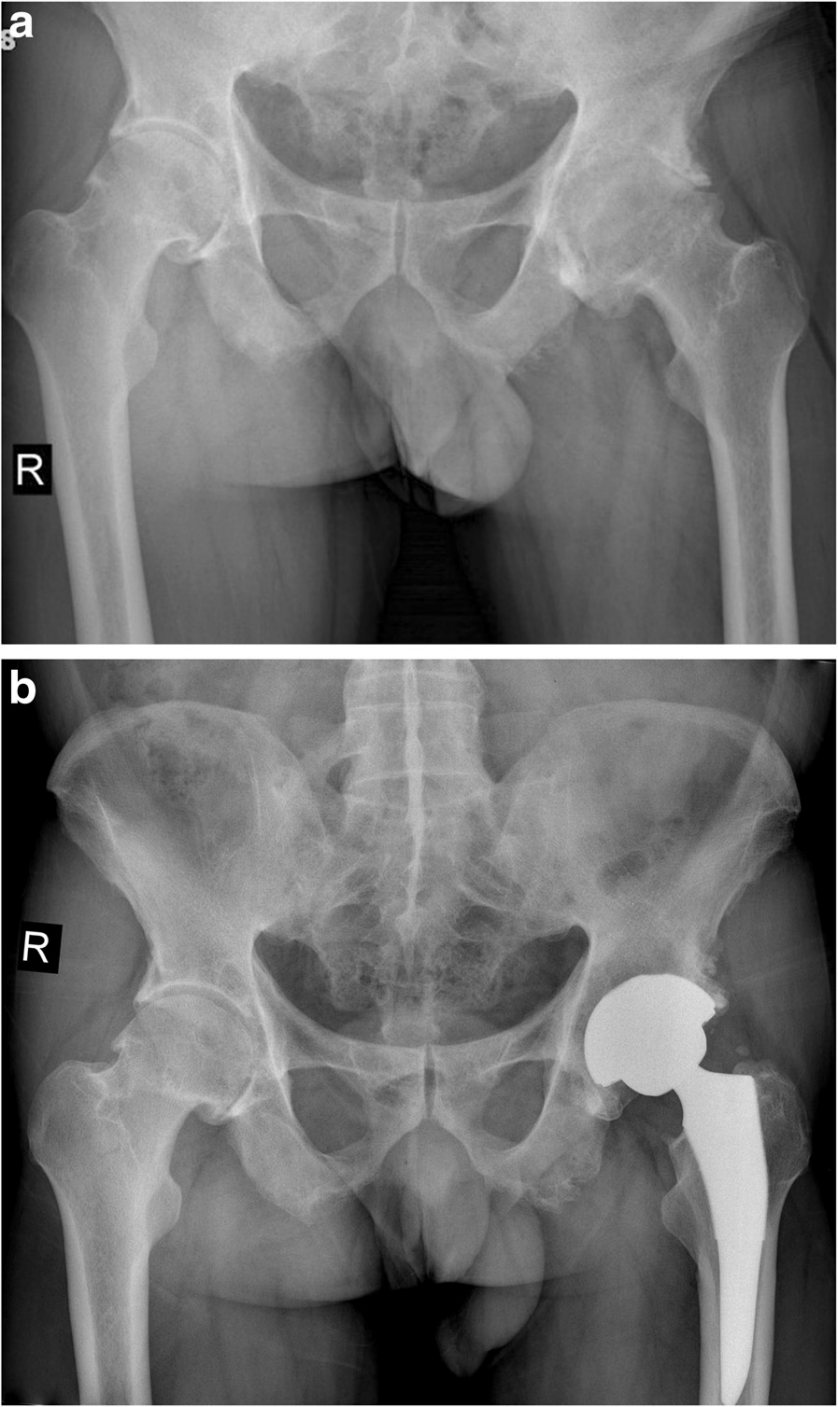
a A preoperative radiograph of the hip of a 73 year-old man who had left hip osteoarthritis. b A radiograph at 36 month after implantation of short cementless femoral stem showed solid fixation in a satisfactory position
